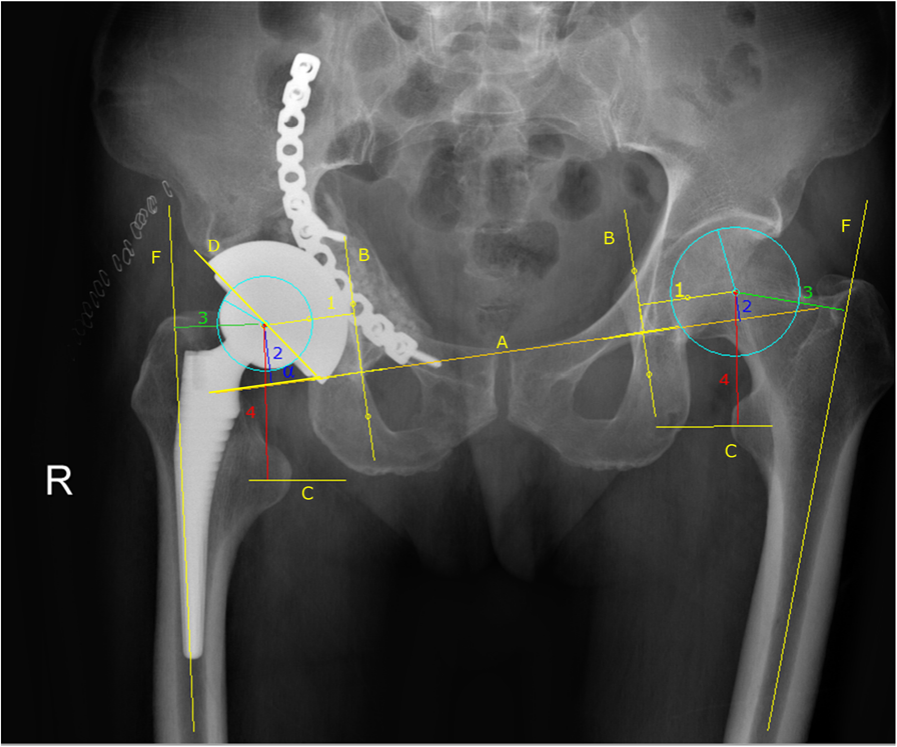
Postoperative radiograph after THA with impacting bone grafting combined with a cementless cup. A, horizontal teardrop line; B, vertical teardrop line; C, midline lesser trochanter; D, a line along the lateral surface of the acetabular component; F, femoral shaft line. 1, horizontal hip center of rotation; 2, vertical hip center of rotation; 3, horizontal femoral offset; 4 vertical femoral offset; α, the angle between a line drawn between line A and line D
